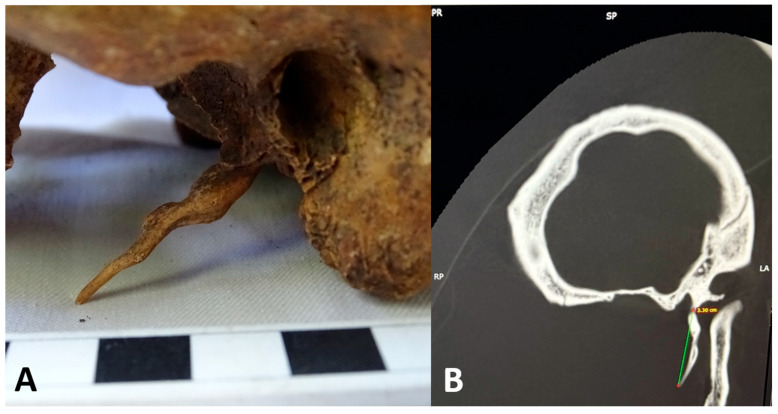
Case No. 2. (A) Left lateral close-up view highlighting the elongated left styloid process (detailed view). (B) Multiplanar CT reconstructions showing linear measurement of the left styloid process.
